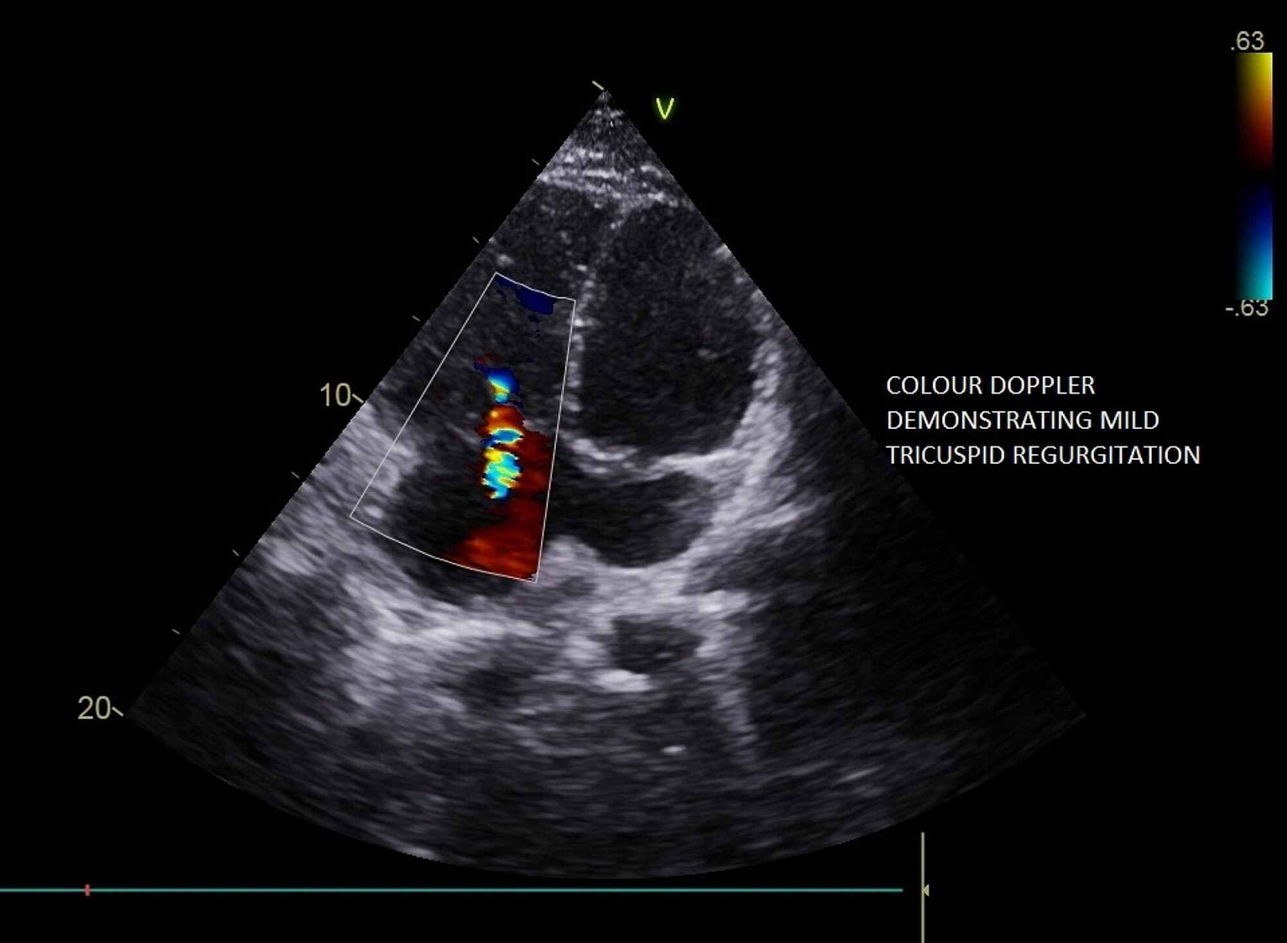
Color Doppler showing mild tricuspid regurgitation
